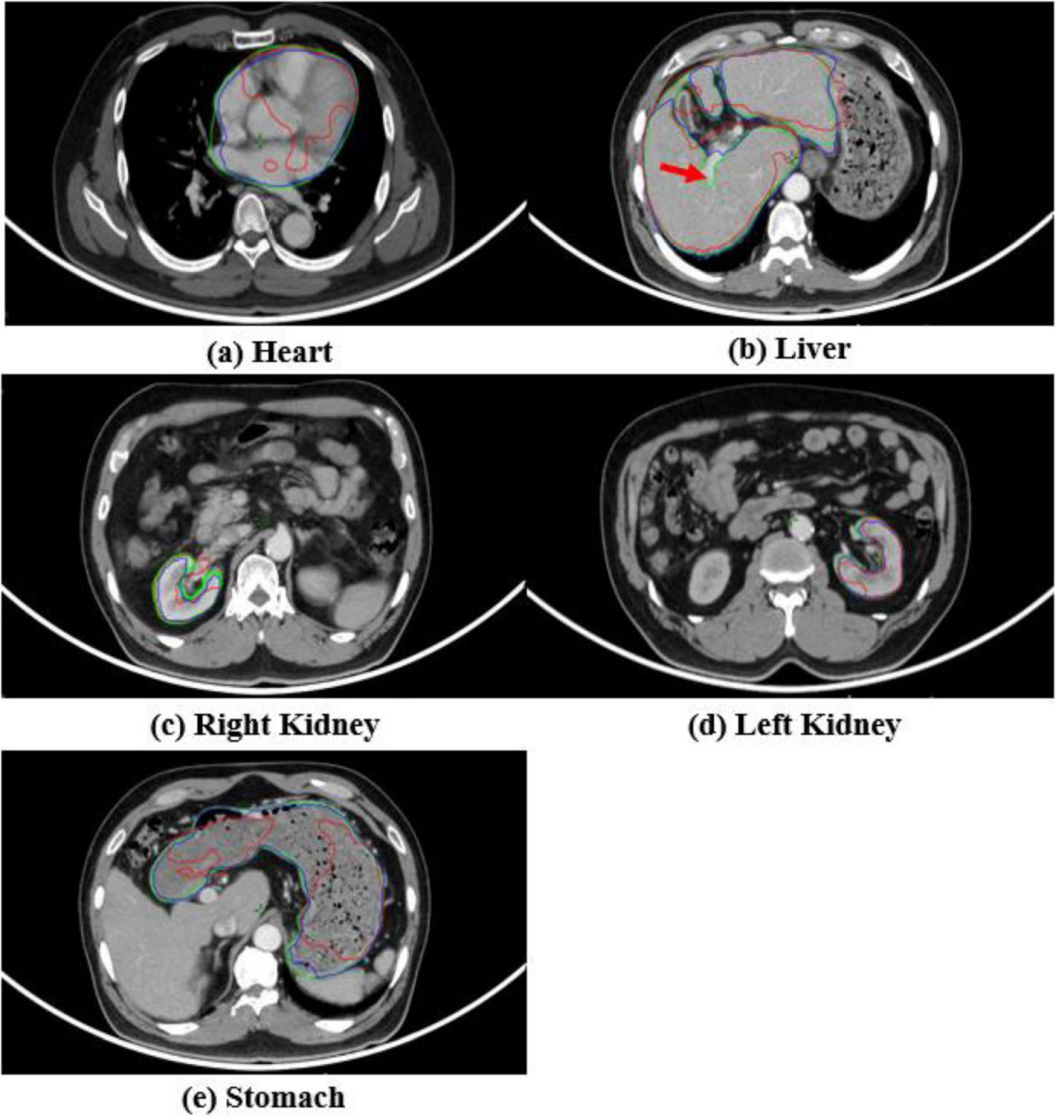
Selected CT slices of one of the studied patients with a manual contour (green), atlas-based contour (red), and deep-learning-based contour (blue) for the (a) heart, (b) liver, (c) right kidney, (d) left kidney, and (e) stomach
